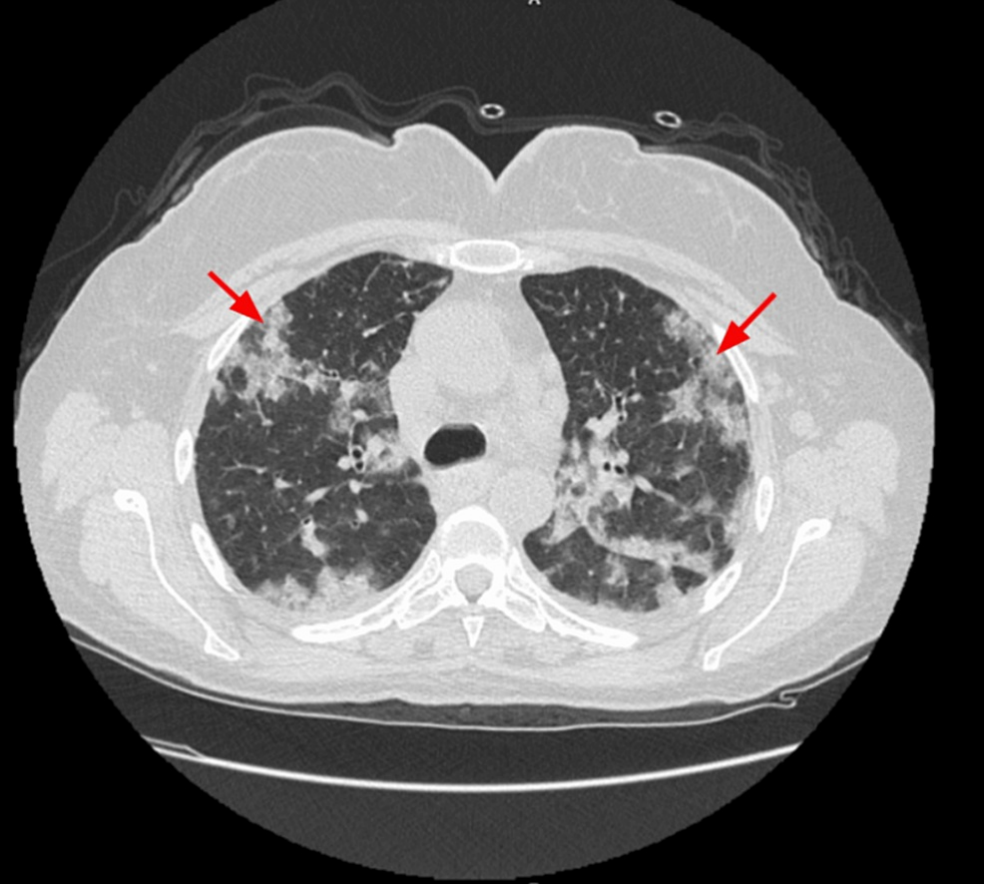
HRCT of the chest HRCT of the chest showing wide areas of patchy infiltrates in multiple areas within the lung parenchyma (red arrows). These findings alongside the patient’s presentation are suggestive of hemorrhage in the lungs.
